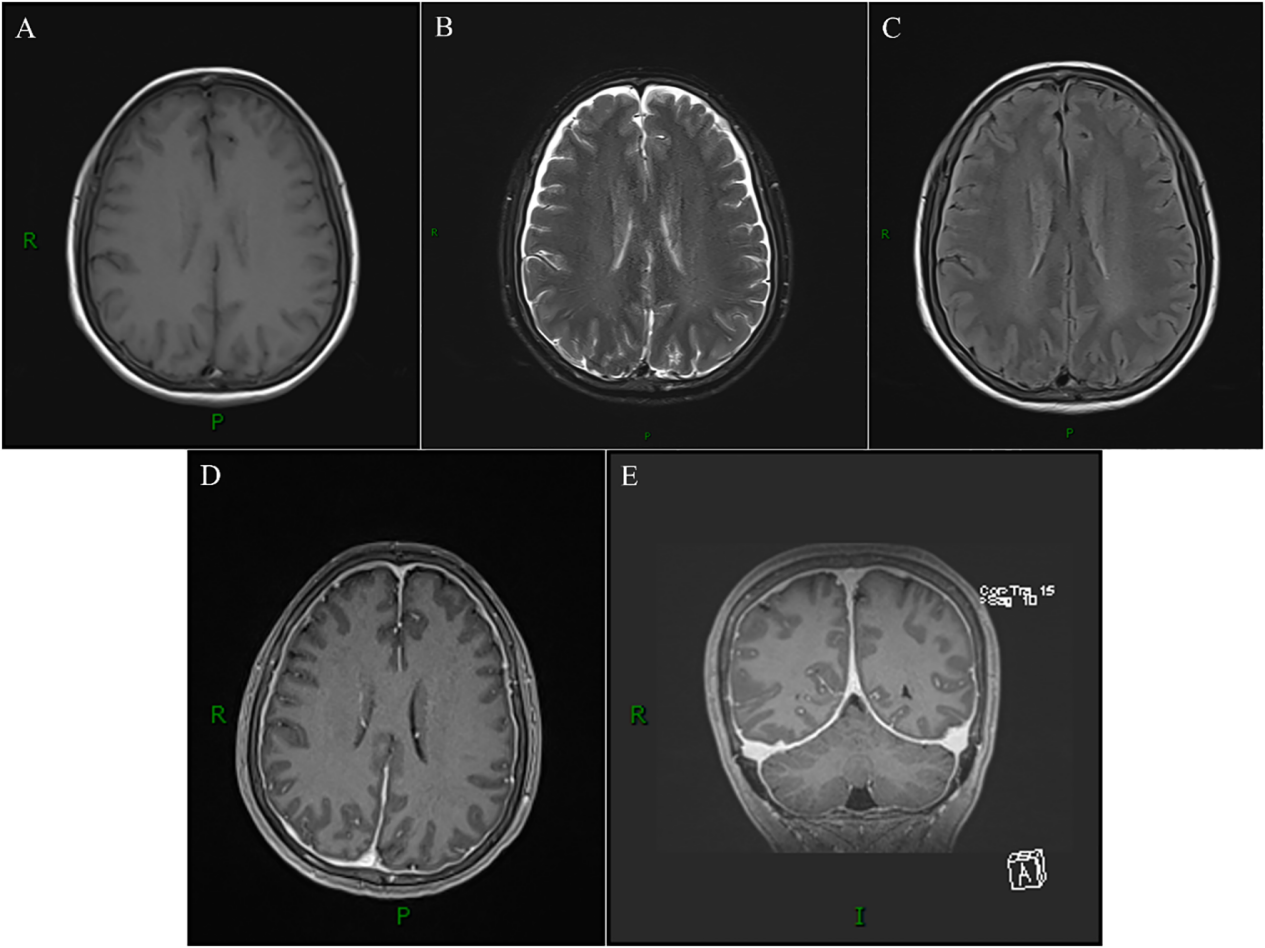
Post-thoracentesis brain MRI (non-contrast + contrast) from April 2023 demonstrates bilateral subdural fluid collections (B), diffuse pachymeningeal thickening with enhancement (D, E), and dilation of bilateral transverse sinuses (E).
